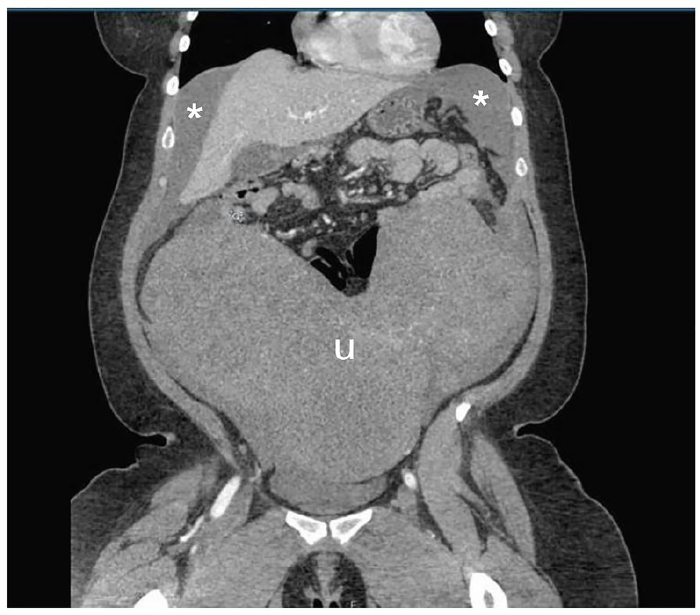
Abdominal computed tomography, coronal view, demonstrating massive fibroid filled uterus (u) and hemoperitoneum (asterisks).
